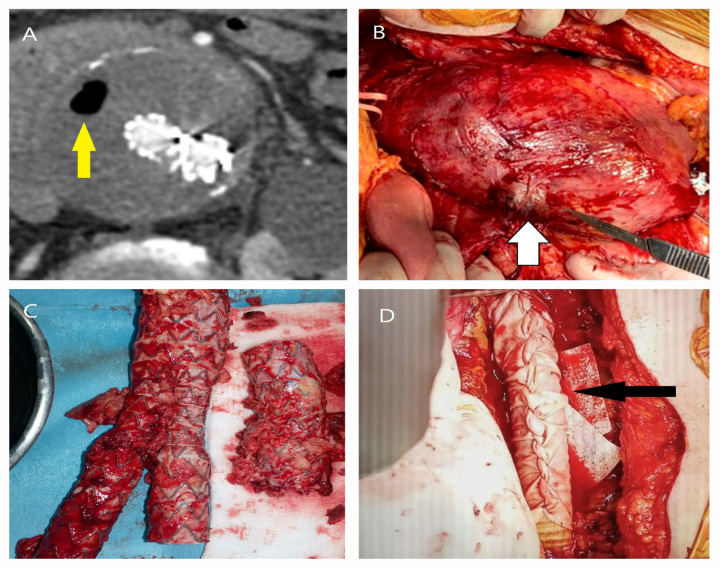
CT and intraoperative images of stent graft infection following EVAR. A 63-year-old male, who underwent EVAR four years ago, was admitted with severe back pain and fever. Blood cultures confirmed Enterococcus faecalis infection. (A) CT showed air (yellow arrow) within the aneurysm sac, indicating infection. (B) Surgery revealed an inflamed aneurysm sac and an infected protrusion (white arrow). (C) The infected stent graft (with iliac limb separation) was removed after proximal occlusion with a balloon. (D) A silver-coated, antibiotic-impregnated Dacron graft was placed and wrapped with a pericardial patch (black arrow) and omentum.
